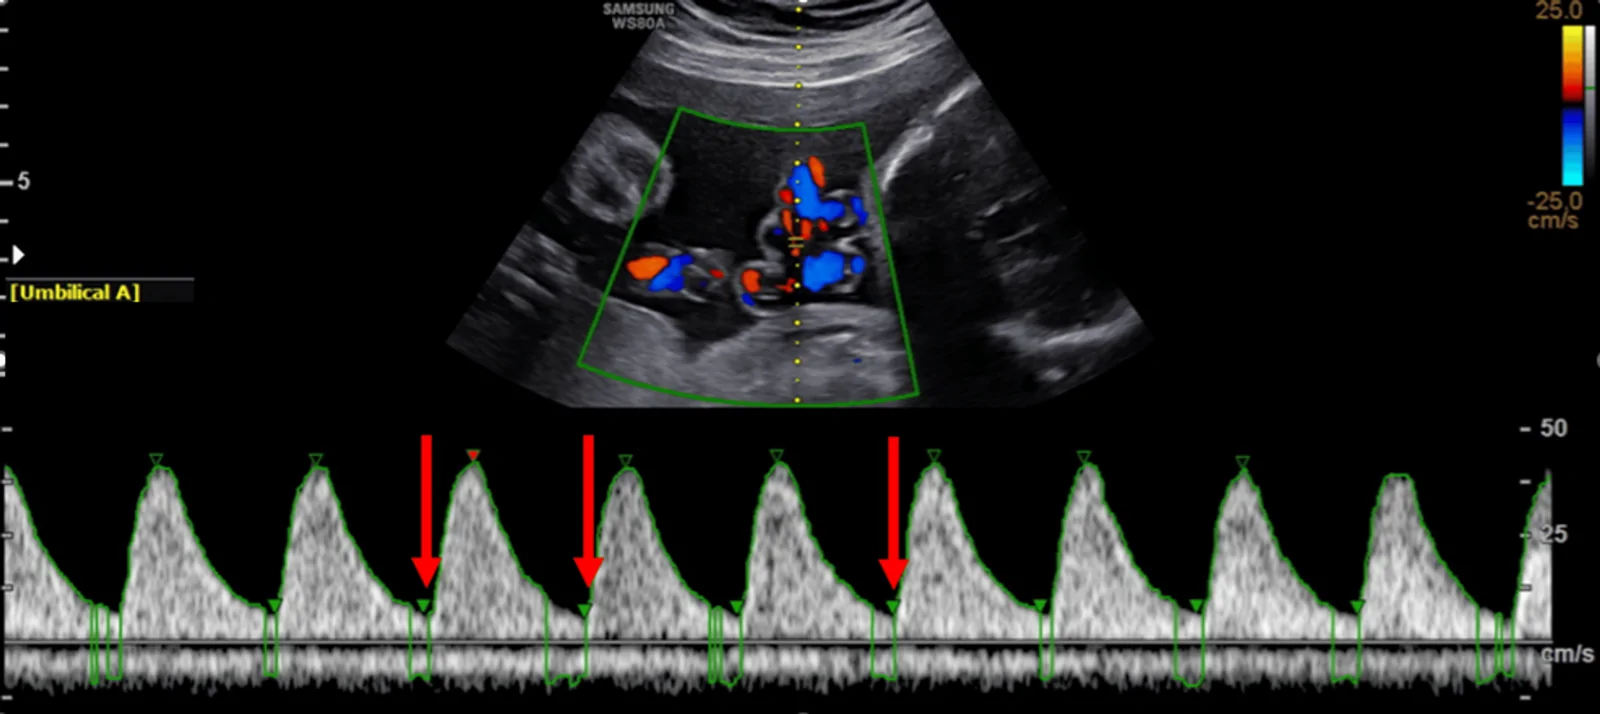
Umbilical artery Doppler ultrasound demonstrating reduced end-diastolic flow. The arrow indicates reduced end-diastolic flow within the umbilical artery waveform. The pulsatility index (PI) of 1.69 is above the 95th centile for 30+3 weeks' gestation, indicating increased placental vascular resistance consistent with placental insufficiency.
